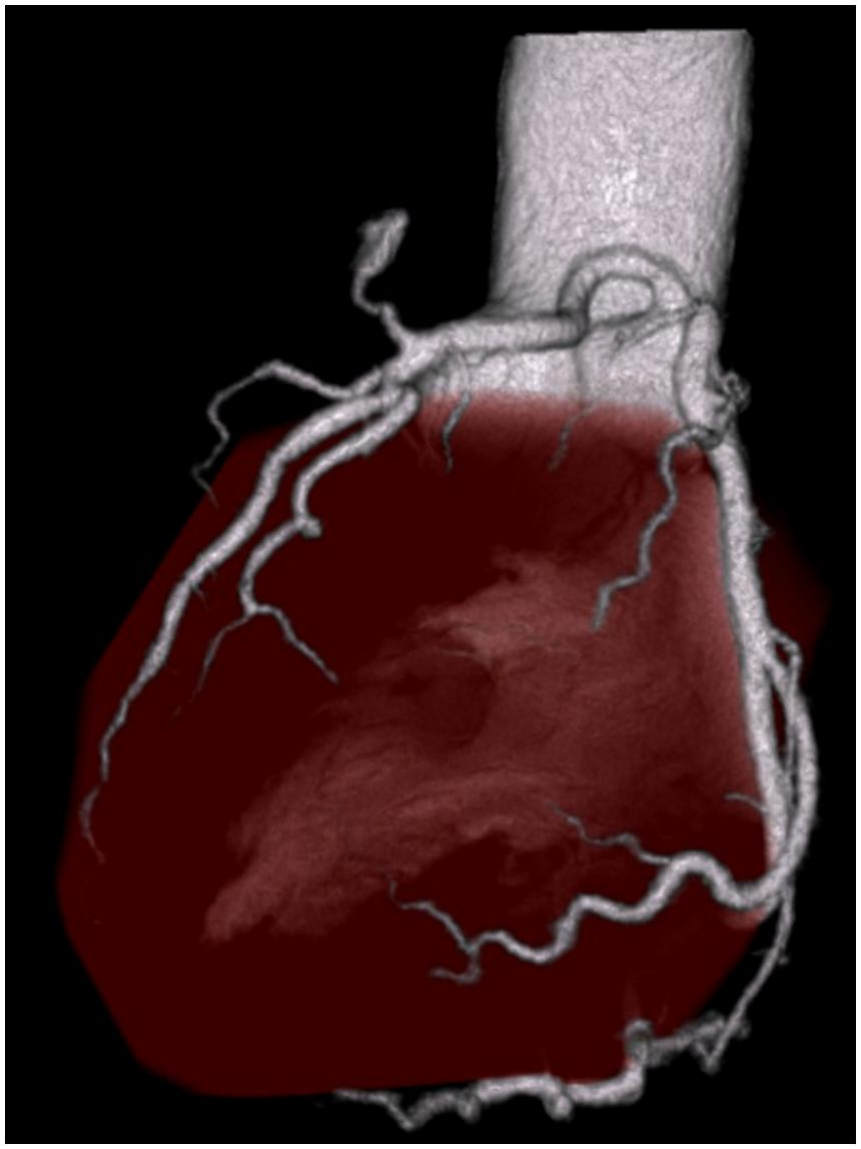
Postoperative CT. CT; computed tomography.
